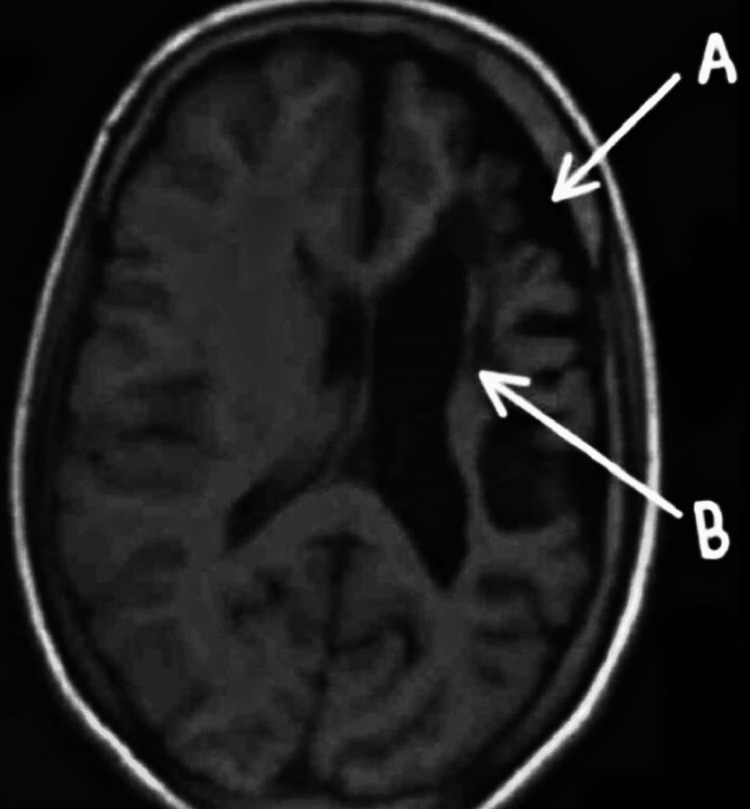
A: T1-weighted axial MRI reveals atrophy in the left frontoparietal and temporal areas of the brain. B: Dilatation of the left lateral ventricle
